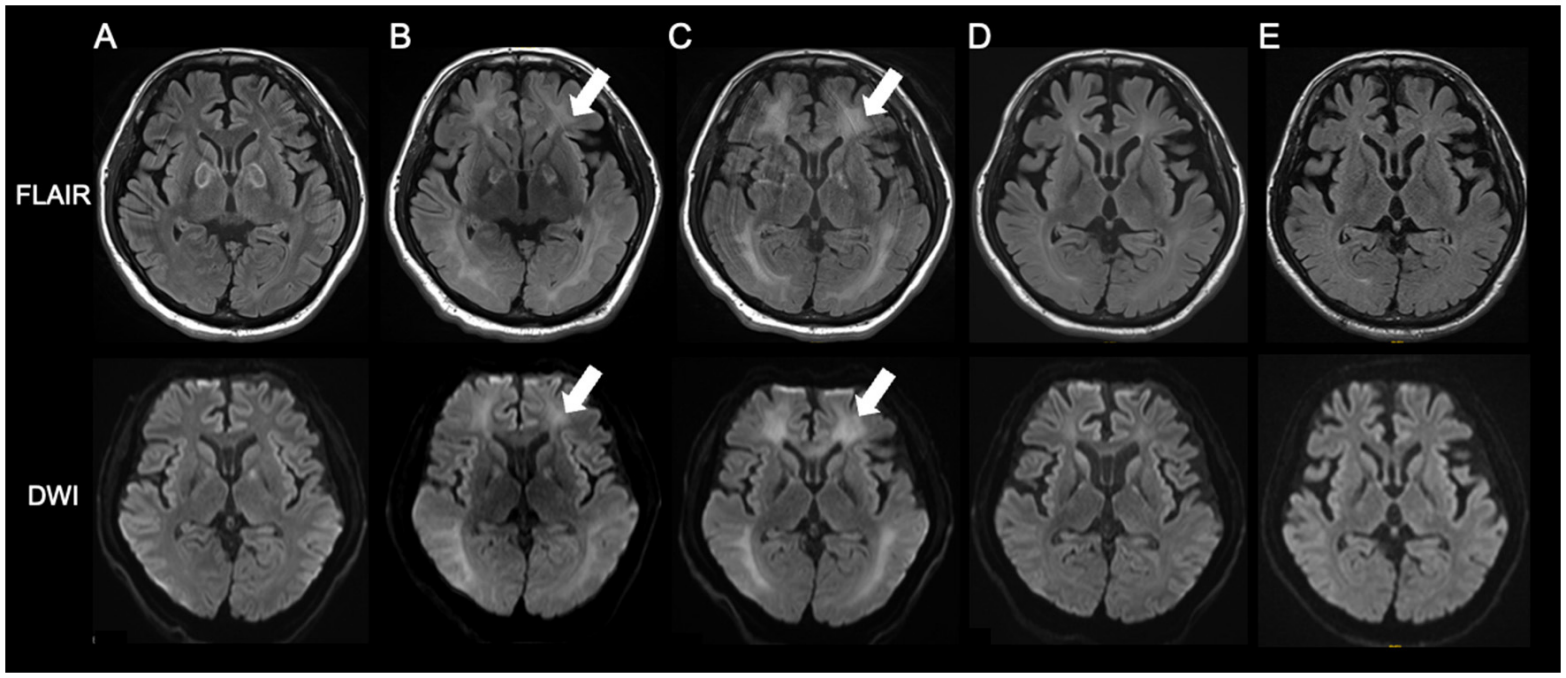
The course of MRI findings. (A) day 11; (B) day 25; (C) day 38; (D) day 89; (E) day 165. White matter abnormalities become apparent from day 25 and gradually disappear after consecutive HBO therapy. HBO, hyperbaric oxygen; MRI, magnetic resonance imaging.
